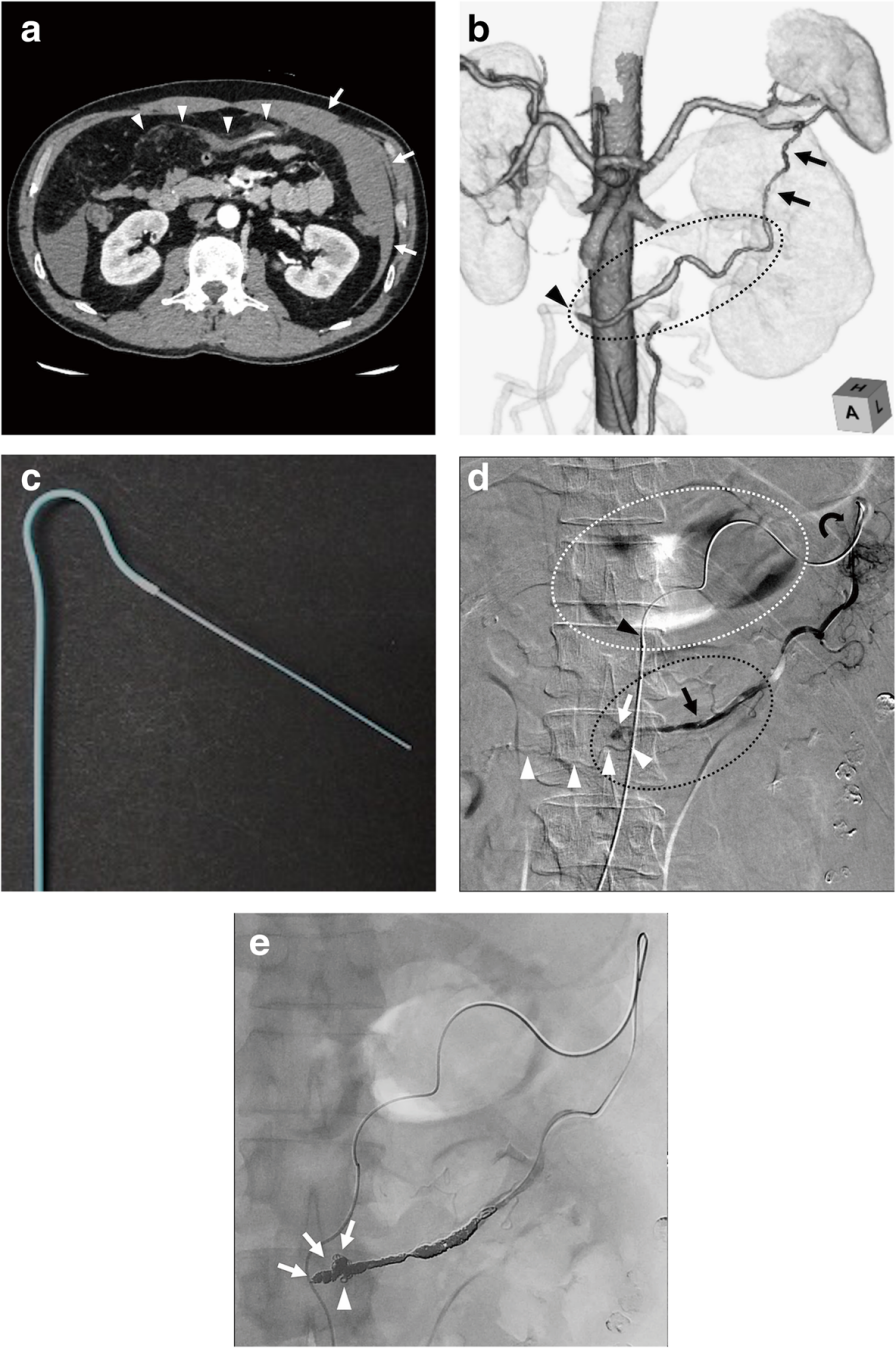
Images from a 55-year-old man with left omental artery (OA) bleeding associated with segmental arterial mediolysis (SAM). a Axial contrast-enhanced computed tomography (CT) reveals massive intraperitoneal high-density ascites mainly involving the left portion of the greater omentum (arrows) where the OA with the dilated, stenotic change develops the possible hematoma (arrowheads). b Coronal volume-rendered image from CT in the cranial and left anterior oblique projection reveals that the left OA (arrows) arising acutely from a splenic artery branch and coursing away from the greater curvature develops dilated, stenotic change (dotted circle) and that the potential bleeding site at the distal portion of left OA (arrowhead) is far from celiac artery. SAM is the most likely diagnosis, and transarterial embolization is planned. c Photograph of the triaxial catheter system consisting of a 4.2 Fr shepherd’s hook catheter, a 2.8 Fr high-flow microcatheter and a 2.0 Fr microcatheter, which serves as the outermost, intermediate, and innermost catheters, respectively. d Digital subtraction angiography (DSA) of the left OA in posteroanterior projection using the triaxial catheter reveals a pseudoaneurysm in the distal portion (white arrow). Note an intact distal left. OA runs through (white arrowheads). Coil embolization is planned to isolate the proximal and distal artery (black dotted circle). Note that the SAM-associated left OA courses away from the greater curvature (white dotted circle), which means that this artery differs from left gastroepiploic artery. Note each tip of the triaxial catheter system consisting of the 4.2 Fr shepherd’s hook catheter (black arrowhead), the 2.8 Fr high-flow microcatheter (black curved arrowhead) and the 2.0 Fr microcatheter (black arrow). e DSA of the left OA in posteroanterior projection after embolization reveals complete hemostasis. Note that distal omental artery including the pseudoaneurysm (arrows) is densely embolized and that the distal normal left OA is also embolized with metallic coils (arrowhead)
